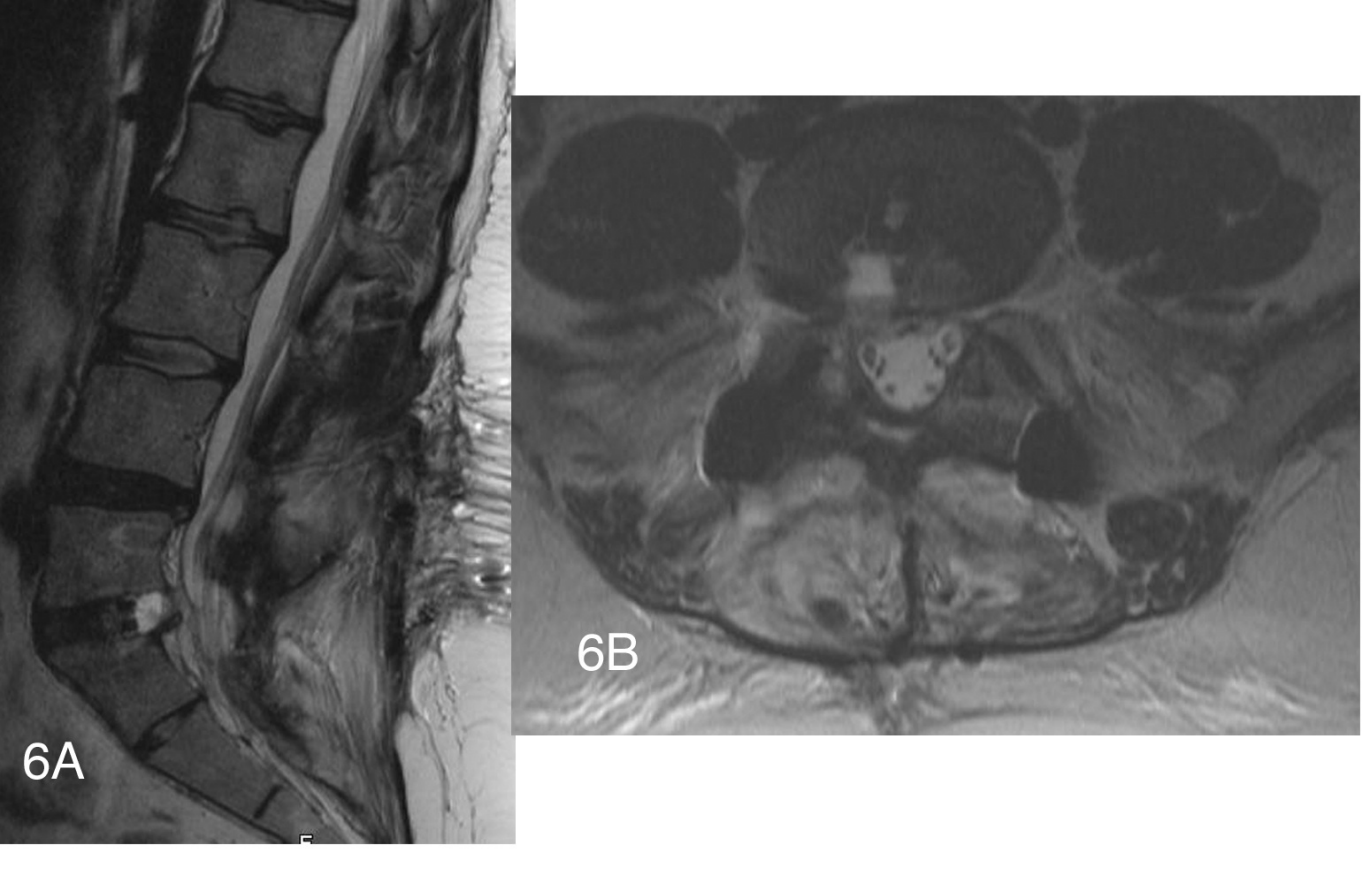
Postoperative T2 weighted sagittal and axial MRI imaging showing cyst T2 weighted sagittal and axial MRI imaging demonstrating right sided cystic  flud collection in the disc space. The right S1 nerve root appears to be displaced posteriorly on the sagittal image.
